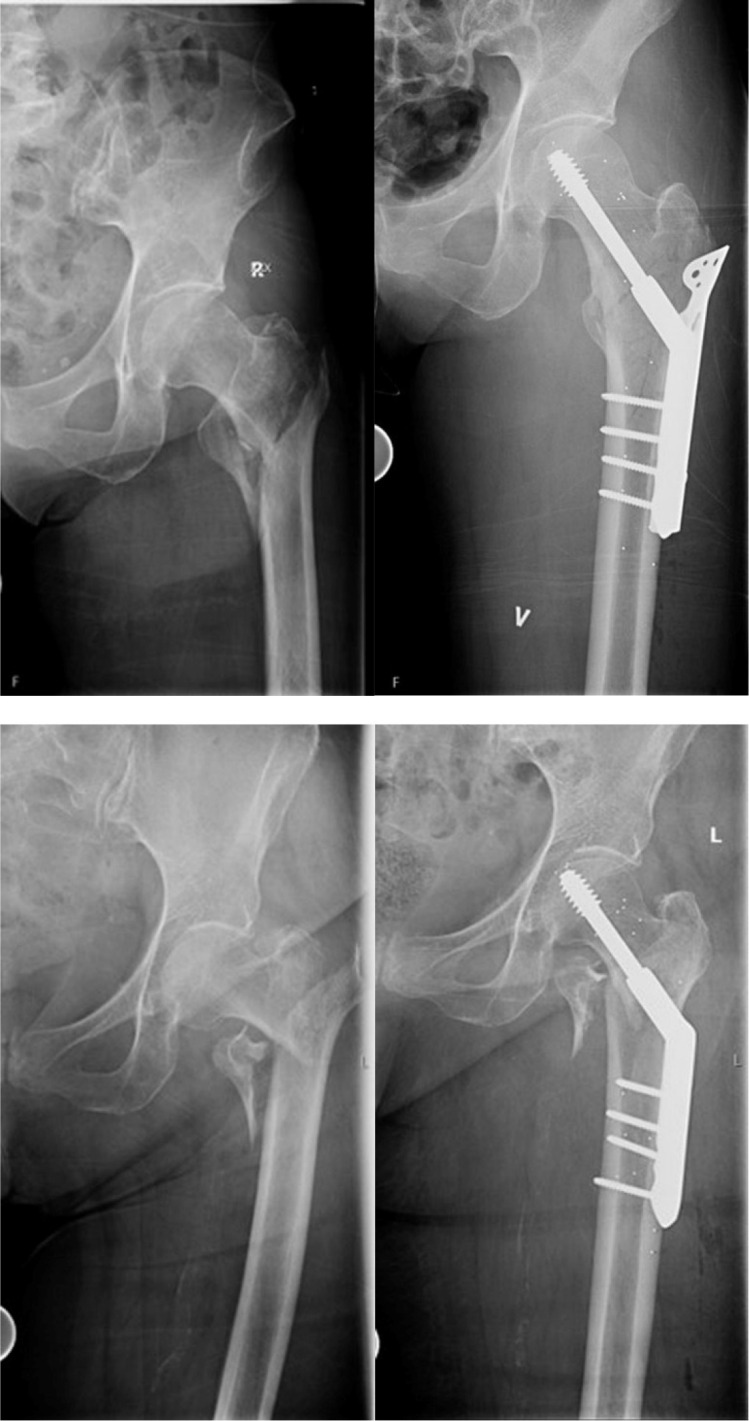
Examples of included AO/OTA 31-A2 fractures. Pre- and postoperative radiographs.
